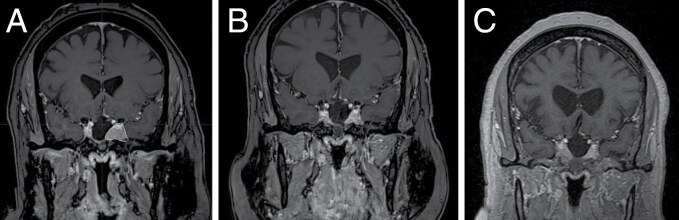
(A) Procedural, T1-weighted coronal image of a 64 year-old woman that presented with a progressive residual nonfunctioning PitNET involving the left cavernous sinus. She underwent single-session Gamma Knife radiosurgery, delivering a prescription dose of 15 Gy (yellow line) in a single fraction. (B) Radiographic tumor regression was noted at the 5-year follow-up. (B) Tumor control was maintained 10 years after the radiosurgical treatment.
